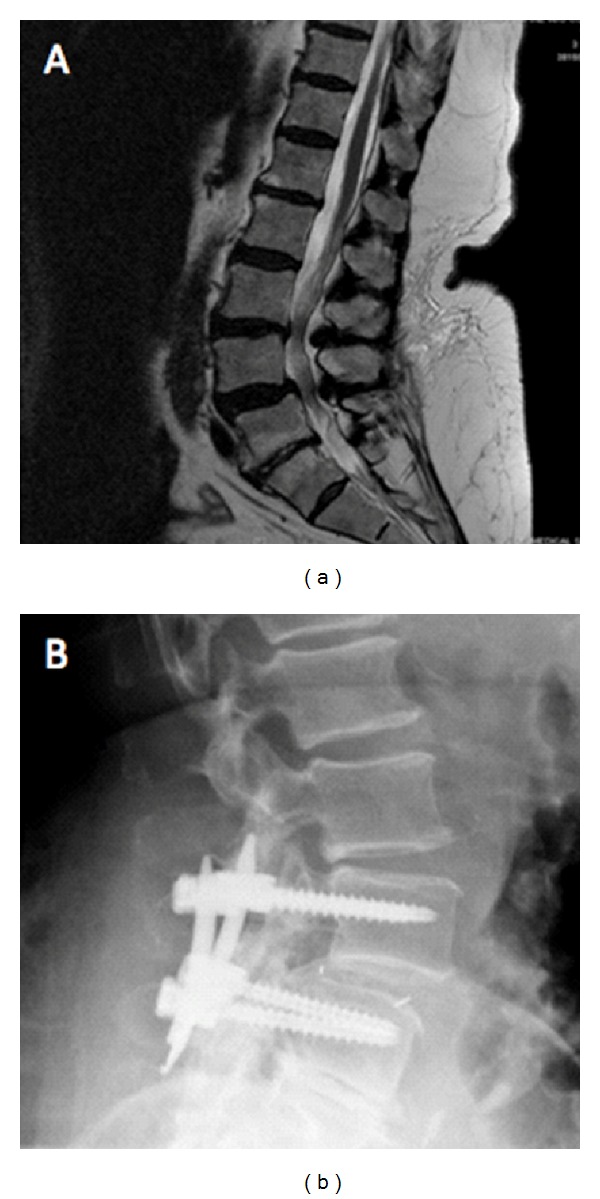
(a) Preoperative lateral MR image of a 72 y/o female patient with back and left leg pain and L4/L5 spondylolisthesis; (b) post-operative lateral MR image from a patient who underwent an MI-TLIF for spondylolisthesis at L4/L5.
